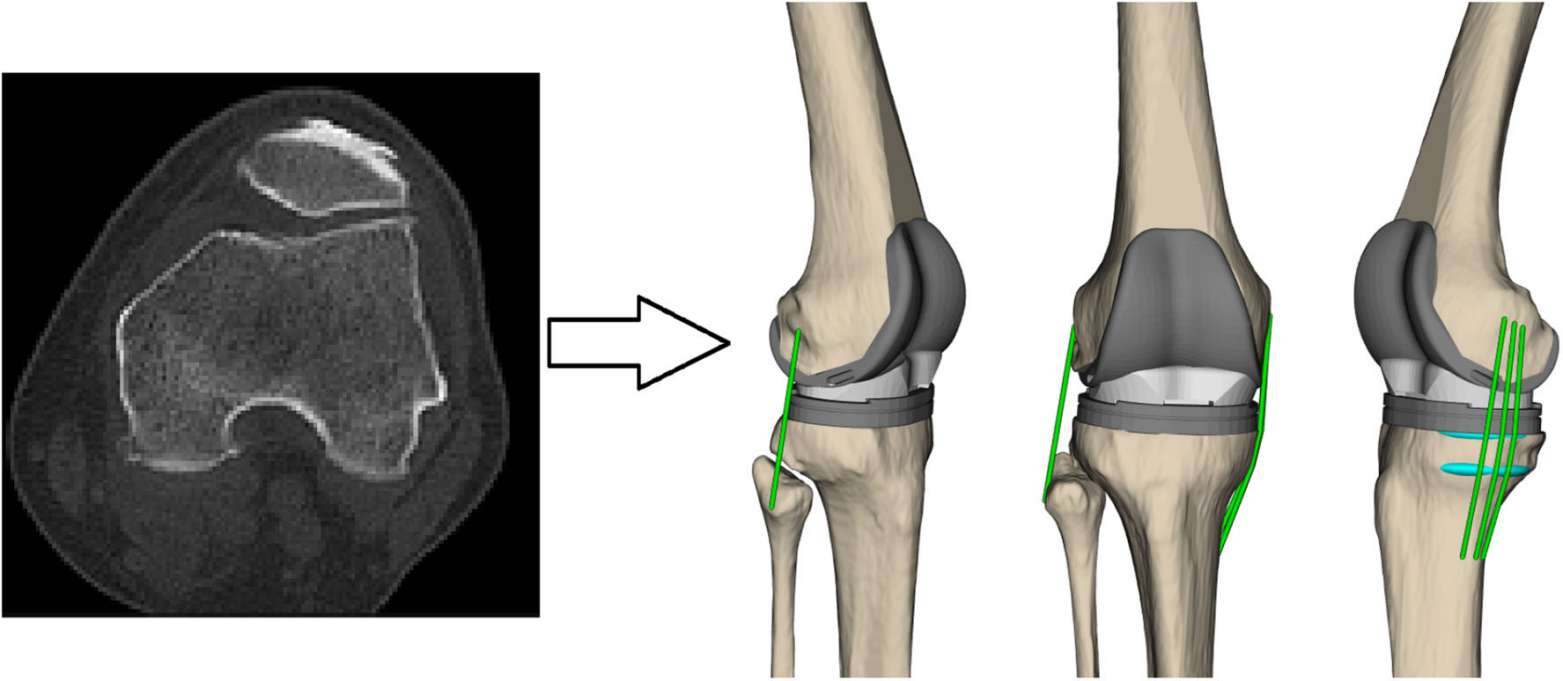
An exemplary pre-operative CT slice (left) together with a subject-specific multi-body model of the knee (right), including the collateral ligament bundles (shown in green) as well as the wrapping surfaces used to prevent penetration of the ligament bundles into the bones (shown in cyan).
